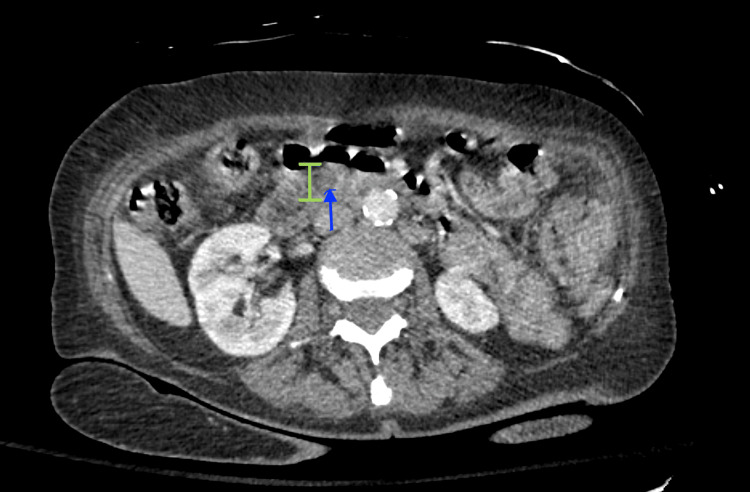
CT scan of the abdomen/pelvis showing increased dilatation of the common bile duct measuring up to 9 mm (green distance marker), and a subtle area of hyperattenuation measuring 5 mm (blue arrow) within the distal common bile duct
